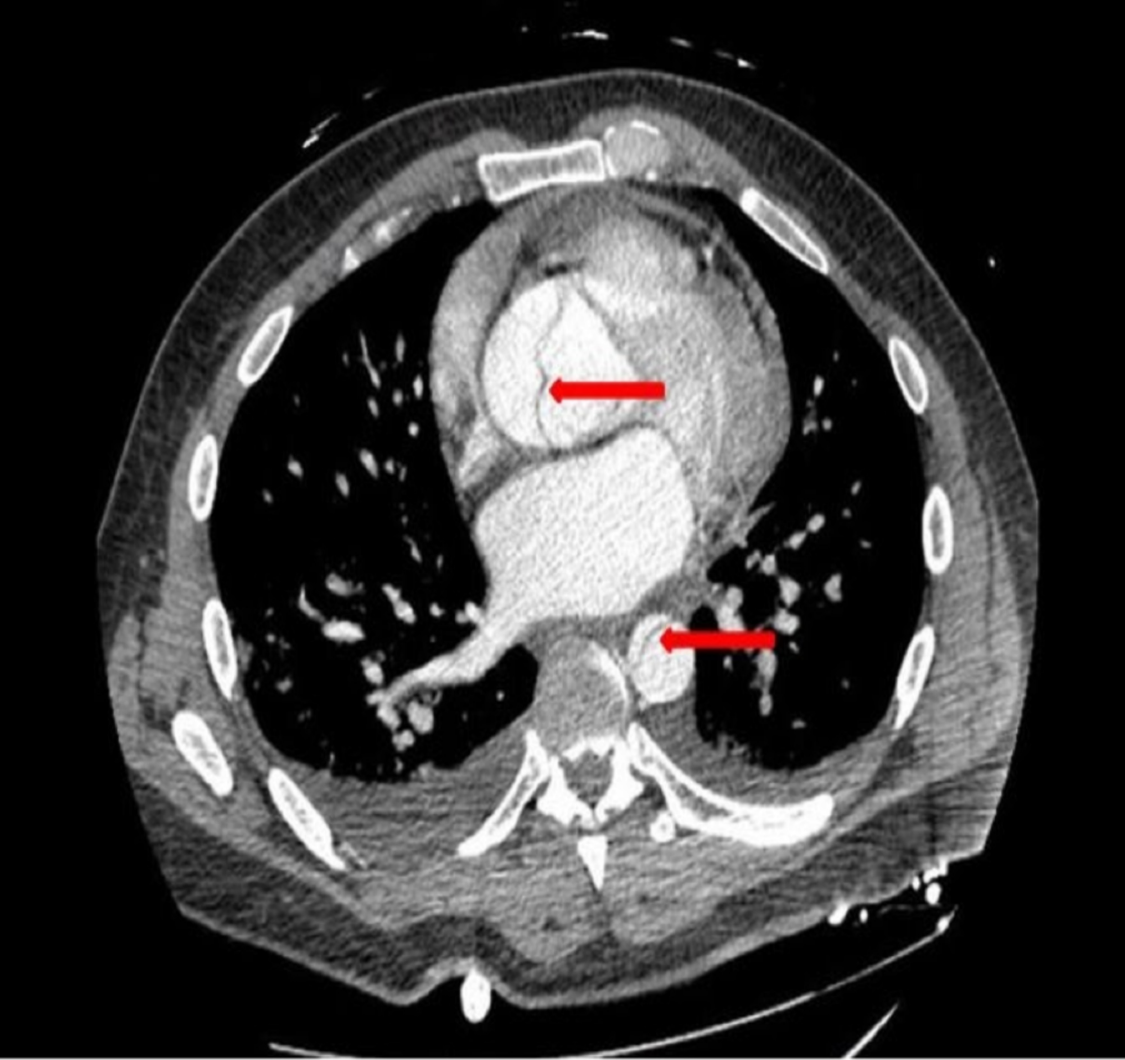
Computed tomography showing evidence of dissection in the ascending and descending aorta as shown by arrows.
